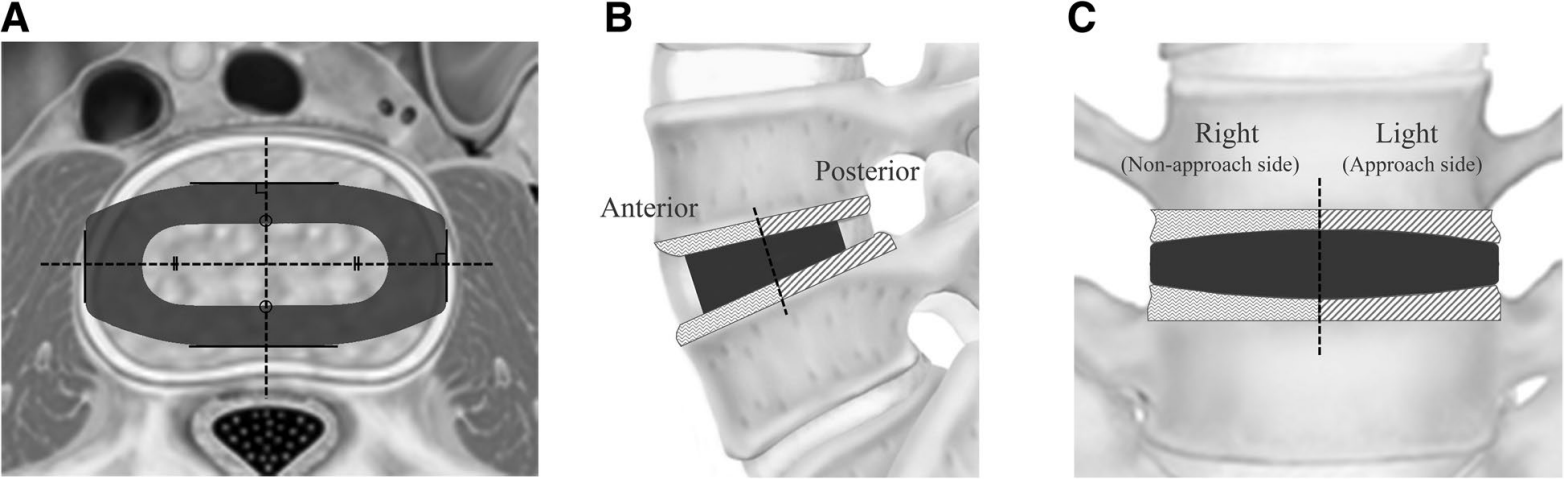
Vertebral area divided by the cage location. Using two-dimensional multiplanar CT reconstructions, CT images were reconstructed parallel to OLIF cage in axial section, and divided into 4 areas based on the center point of the cage (A). Vertically to the cage and parallel to the lines in Fig. 1A, displacement of endplate was evaluated in sagittal section (B) and coronal section (C)
